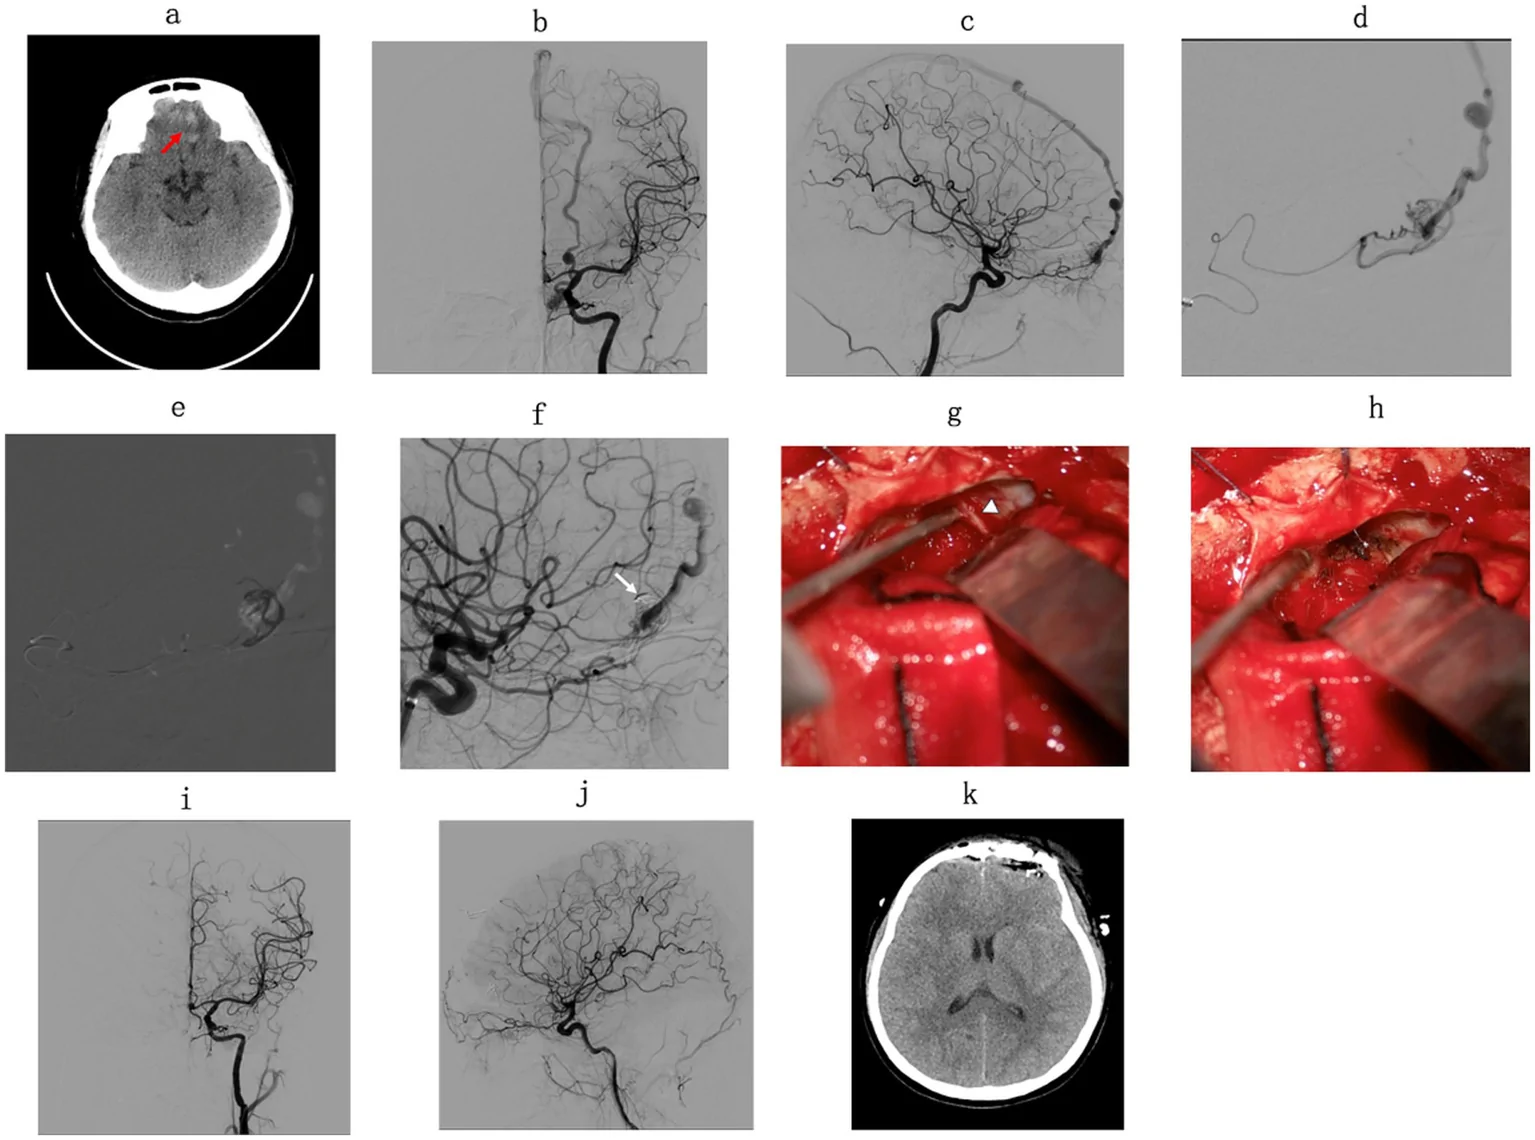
Example of hybrid surgical treatment for anterior cranial fossa (ACF) DAVF. (a) Brain CT reveals an intraparenchymal hemorrhage. (b,c) AP and lateral internal carotid artery (ICA) arteriograms show the ACF DAVF draining into a frontal vein. (d) Selective ophthalmic artery arteriogram shows the fistula draining into a venous pouch. (e,f) Angiograms reveal the Onyx cast and partial embolization of the fistula. (g,h) Intraoperative views of the ACF show the arterialized vein and disconnection of the fistula by coagulation of the draining vein distal to the fistulous point. (i,j) Immediate angiograms demonstrate complete obliteration of the fistula. (k) Postoperative non-contrast axial CT shows embolic material close to the frontal lobe.
